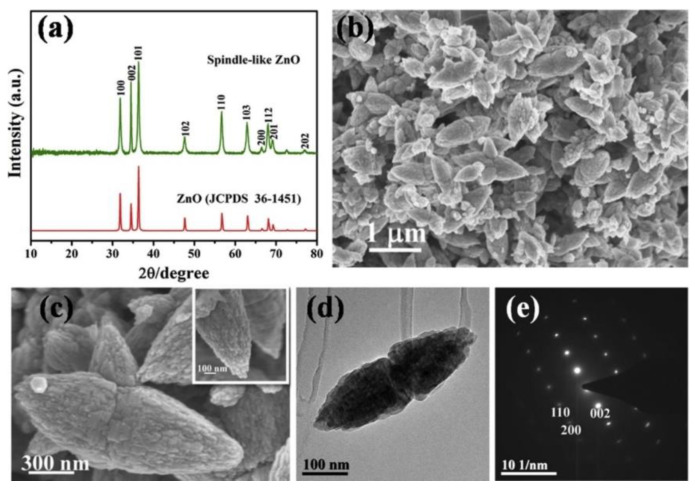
X-ray powder diffraction (XRD) pattern (a), SEM (b,c), TEM (d) images and satisfactory selected area electron diffraction (SAED) pattern (e) of the as-prepared spindle-like ZnO. Products synthesised by microwave and ultrasonic wave combined method. Reprinted from [888], Copyright (2019), with permission from Elsevier [OR APPLICABLE SOCIETY COPYRIGHT OWNER]. All rights reserved. In order to re-use permission must be obtained from the rightsholder.
